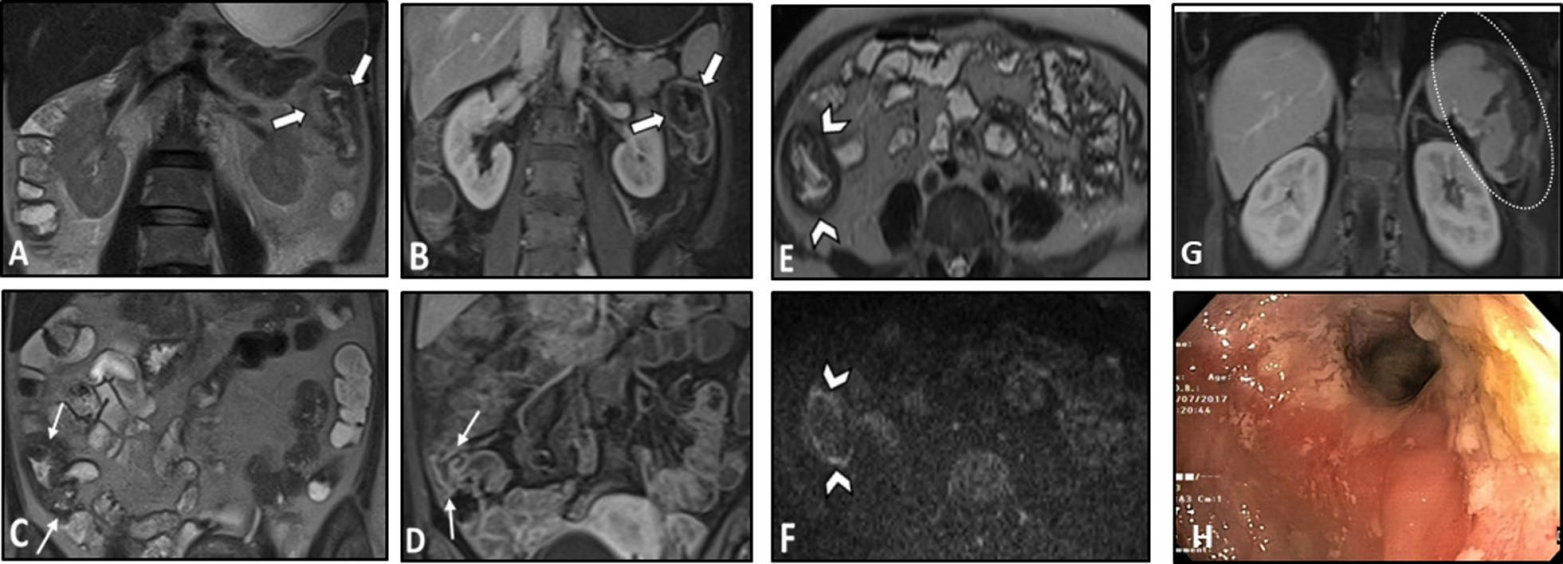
Intestinal ischemia in a 31-year-old female with known SLE-Sjögren overlap syndrome presenting with fever, anemia, and LUQ pain. MRE was obtained. Coronal T2-W and post-contrast T1-W images (A, B) display mural thickening, mucosal hypoenhancement, and serosal hyperenhancement of descending colon adjacent to the splenic flexure (thick white arrows) suggestive for mucosal ischemic changes. Coronal T2-W and post-contrast T1-W images (C, D) display mural thickening and increased mural enhancement of the cecum and ascending colon (Thin white arrows). Axial T2-W image and corresponding DWI sequence (E, F) show concentric mural thickening of the proximal ascending colon, demonstrating restricted diffusion (white arrowheads), in favor of ischemic changes or increased inflammatory cells. Coronal post-contrast T1-W image (G) reveals subcapsular areas of splenic hypoenhancement (white dotted oval), indicative of infarction. Pale and fragile mucosa is seen in colonoscopic view (H) of the involved colonic splenic flexure
